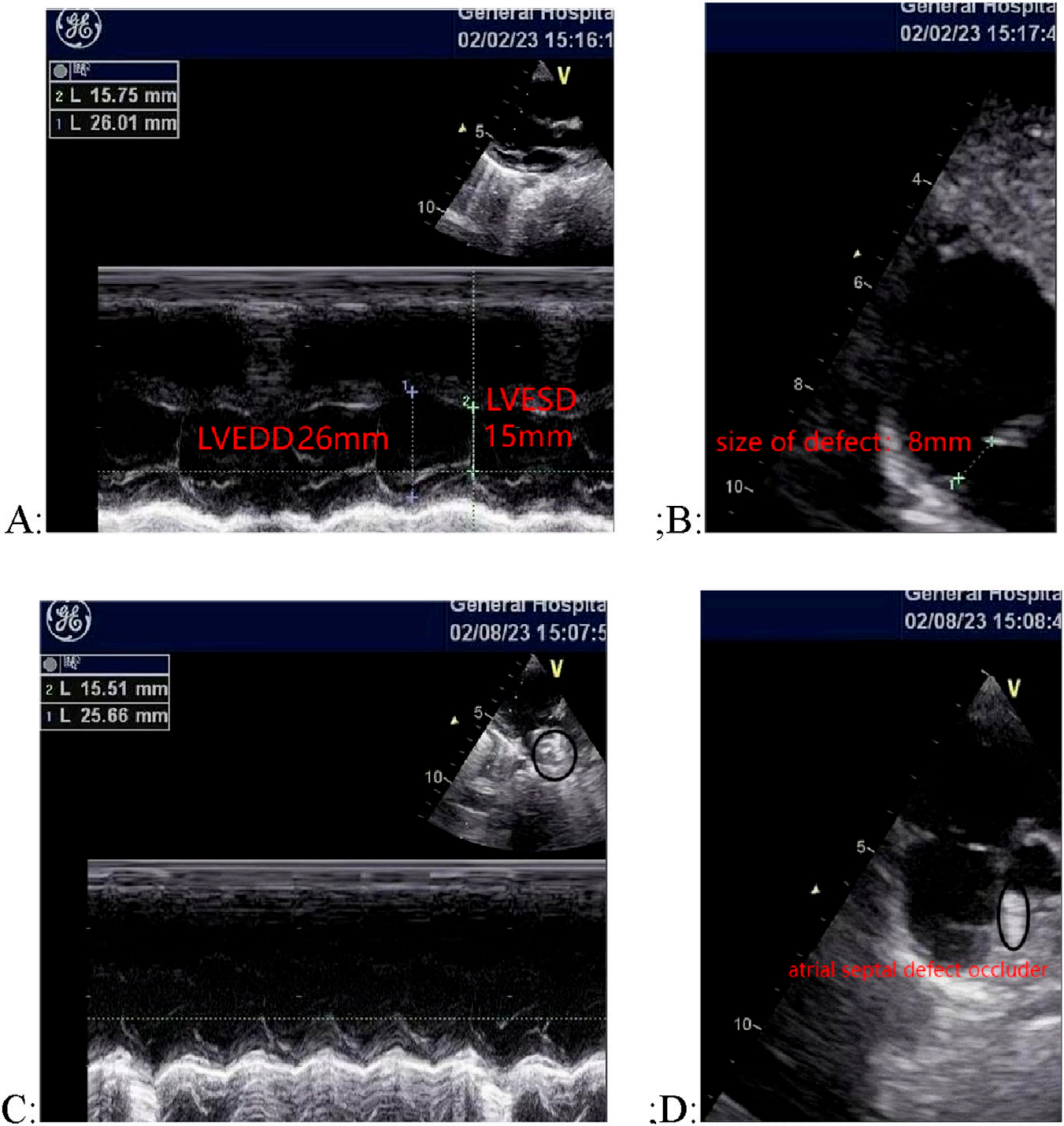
Comparison of cardiac ultrasound images before and after surgery. (A) and (B) Preoperative ultrasound images, showing an 8 mm atrial septal defect; (C) and (D) Postoperative ultrasound images, showing the occluder in place with no shunt or displacement.
